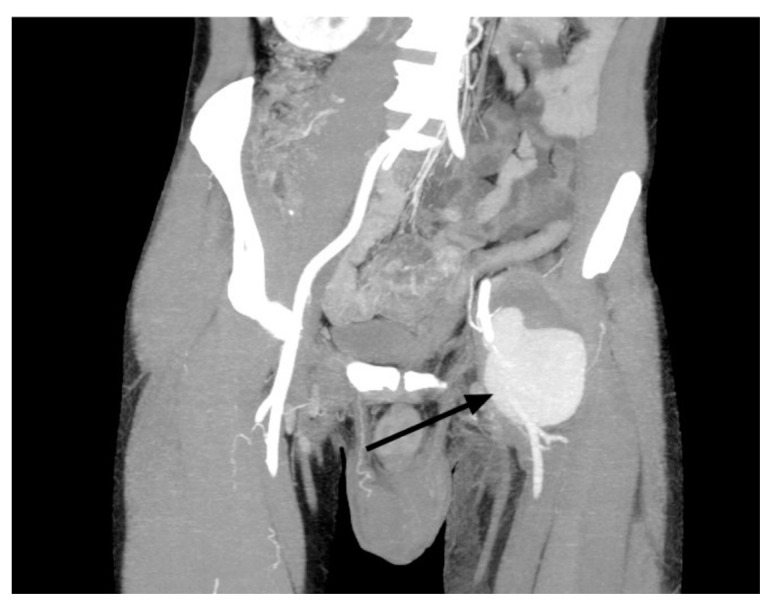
Femoral artery aneurysm in BD. CT angiography demonstrating a saccular aneurysm of the femoral artery (arrow).
